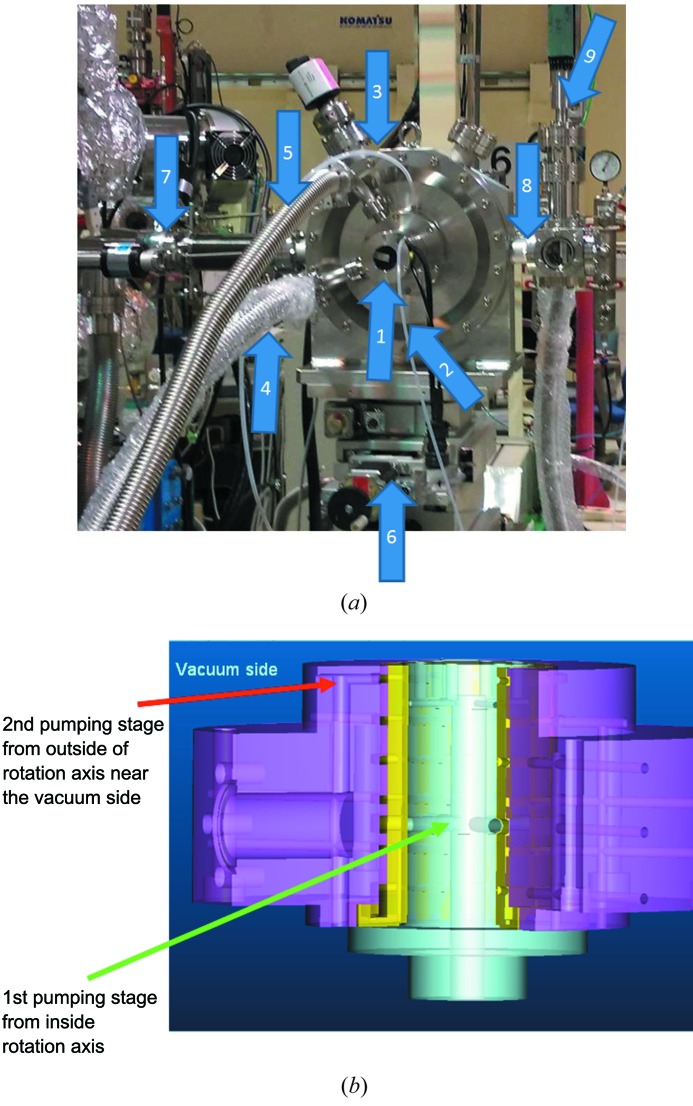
(*a*) Photograph of the air-spindle soft X-ray chopper apparatus. The X-ray beam passes from left (upstream) to right (downstream). (1) Rotation axis. (2) Air inlet for axis cooling. (3) Air inlet for spindle. (4) Pumping port for second stage [see (*b*), turbo-molecular pump]. (5) Pumping port for first stage [see (*b*), scroll pump]. (6) *X–Y*–θ stage. (7) Upstream port, with 5 mm-diameter aperture. (8) Same as (7) but for downstream. (9) Port for PIN photodiode. (*b*) Cross-sectional view of the differential pumping system of the rotational axis. The part shown in light blue is the rotator, and that shown in yellow is the coated and polished wall for the air spindle.
